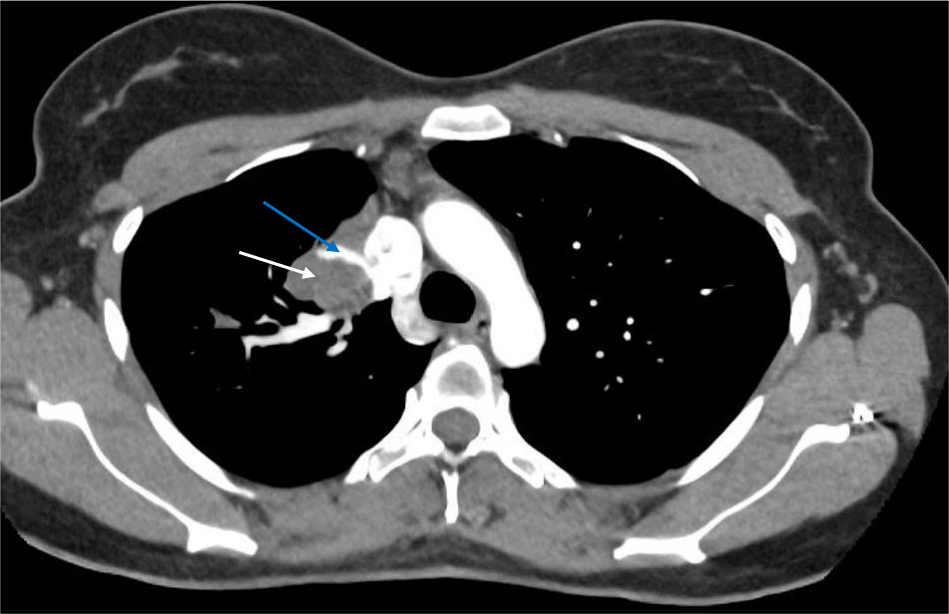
Axial arterial phase CT scan demonstrating pulmonary sequestration (bottom arrow). A large venous branch draining into the azygous vein can be identified (top arrow).
